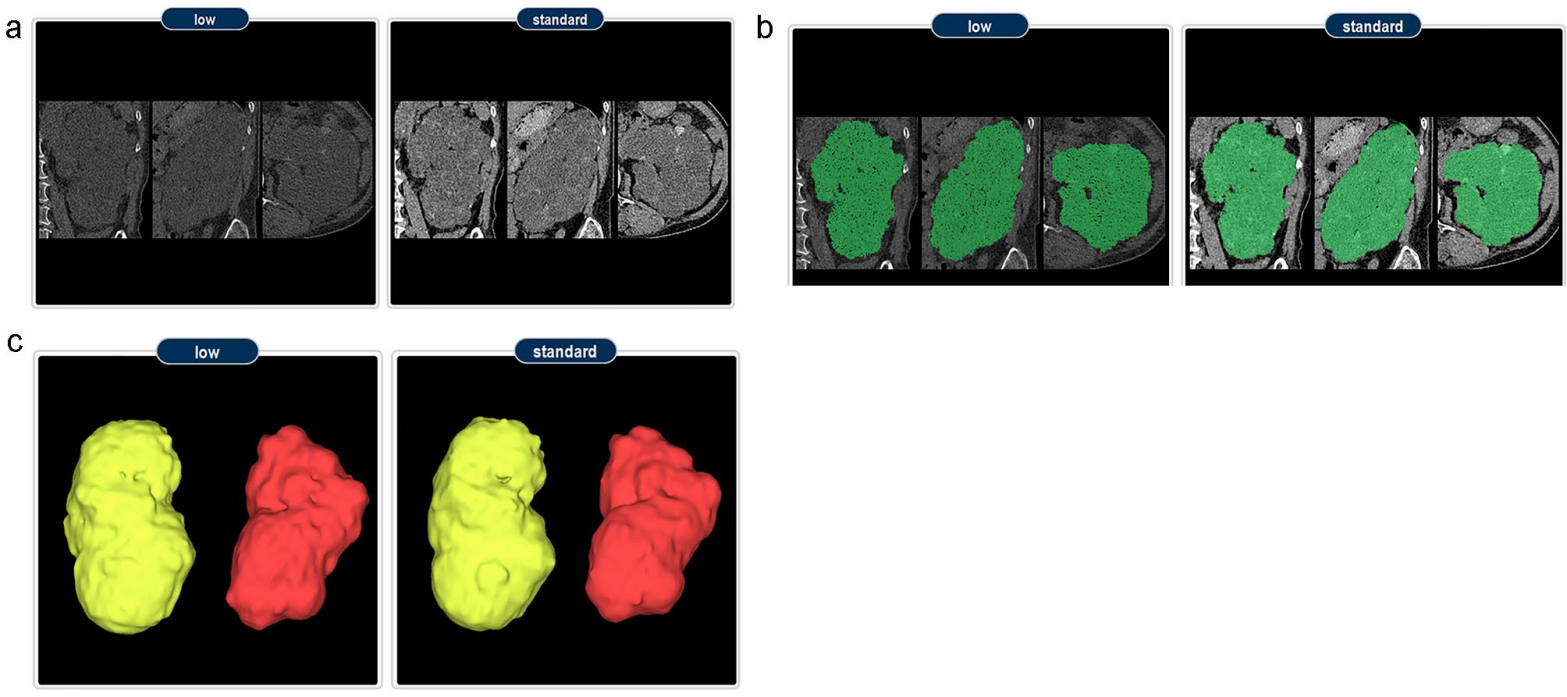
Process of obtaining 3D kidney volume from axial images. (A) CT scans from an ADPKD patient are shown (low-dose CT image and standard-dose CT image, respectively.) (B) Green areas of the kidney segmentation images are the areas identified by the Synapse 3D software as kidney tissue in ADPKD patients. (C) Final 3D reconstructed images of the whole kidneys created by the Synapse 3D software based on consecutive 2D images. 3D, three-dimensional; CT, computed tomography; ADPKD, autosomal dominant polycystic kidney disease; 2D, two-dimensional
